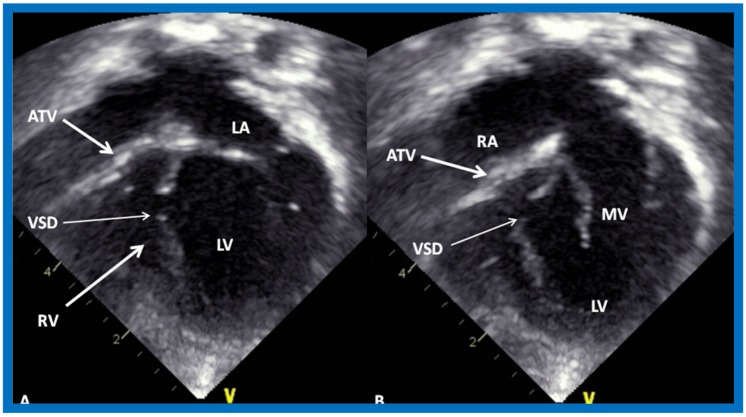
Selected video frames from apical four-chamber, 2-dimensional echocardiographic views of a neonate with tricuspid atresia showing an enlarged left ventricle (LV), a small right ventricle (RV) and a dense band of echoes at the site where the tricuspid valve echo should be (ATV) (thick arrow) with closed (A) and open (B) mitral valve. A moderate sized ventricular septal defect (VSD) (thin arrows) is shown. LA, Left atrium; RA, Right atrium. Reproduced from Rao P.S. [39].
